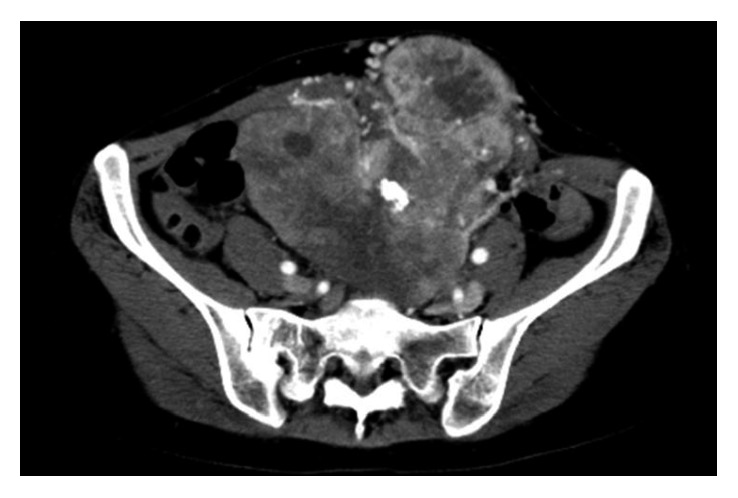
Enhanced computed tomography findings at the initial visit. CT revealed marked dot-like enhancement at the arterial phase, suggesting abundant vascular formation.
